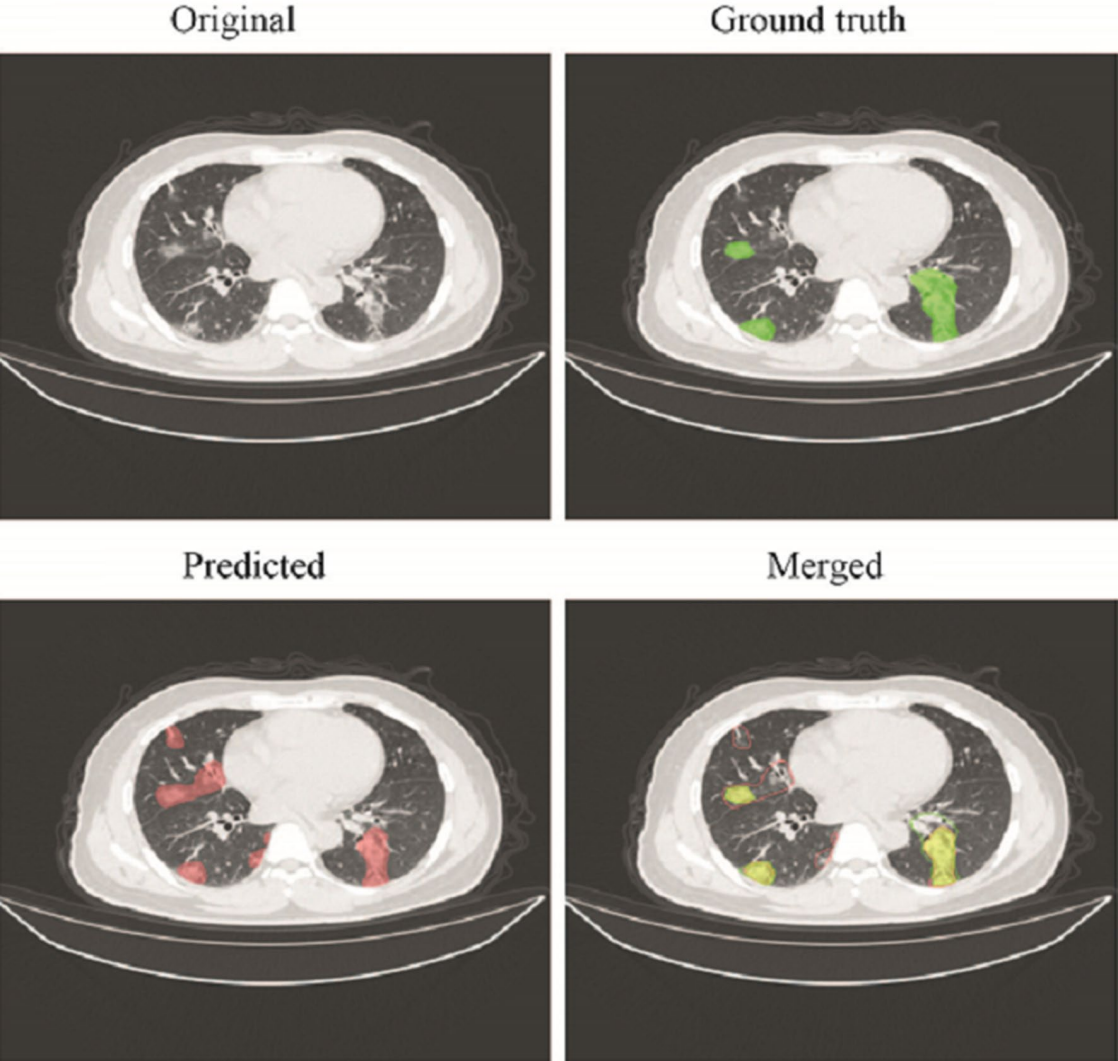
The representative of coarse annotated (Green) and AI labeled (Red) ROIs. Although trained with coarse annotated slices, DL-based segmentation algorithm could recognize and delineate most of the lesions on CT scans in the testing datasets.
